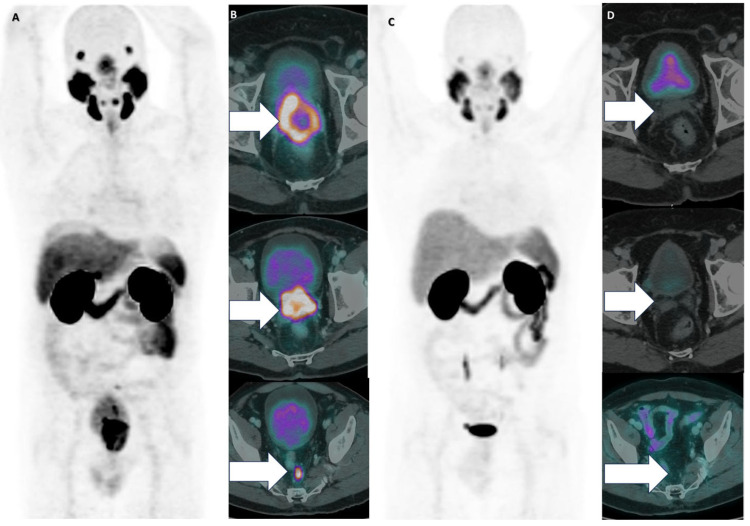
A 65-year-old male with Gleason score 10, plasmacytoid variant, MSI-H/PDL1 prostate adenocarcinoma. The patient was previously treated with goserelin and enzalutamide. A baseline [^68^Ga]Ga-PSMA PET/CT was performed with PSA 14.10 ng/mL (A), MIP. The axial slices with arrows show pelvic LNM and infiltrating prostate mass, locally advanced in large and small bowel and bladder (B), The patient was treated with four cycles of [^177^Lu]Lu-PSMA PRLT. [^68^Ga]Ga-PSMA PET/CT was performed at six months post four cycles of [^177^Lu]Lu-PSMA PRLT (C), MIP. The axial slices with arrows highlight a remarkable complete response in all tumor sites (D). The patient had complete clinical, molecular and biochemical response with no new symptoms for 48 months.
